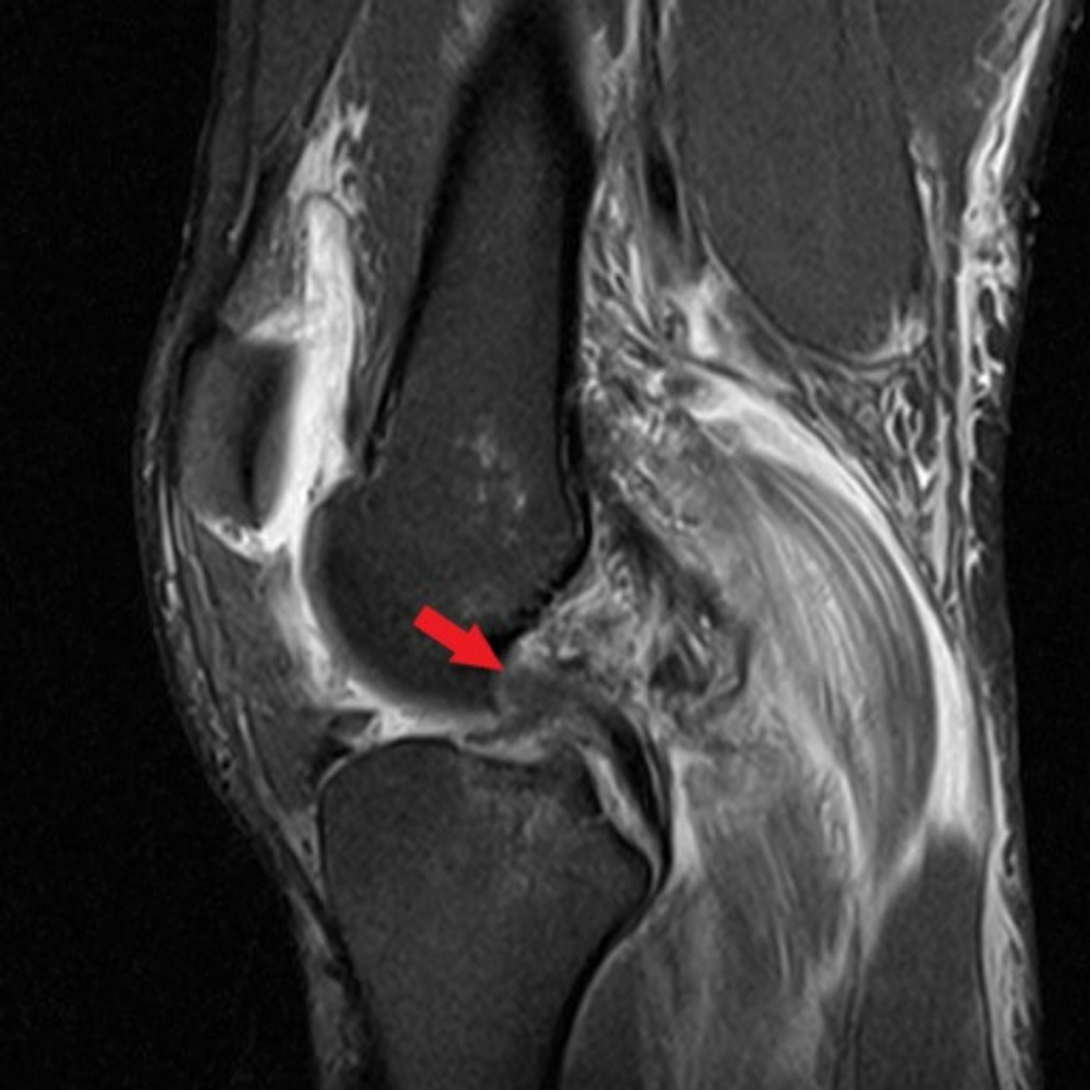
Sagittal T2-weighted MRI of the left knee in patient 2 Sagittal T2-weighted MRI of the left knee demonstrating an increased signal in the proximal aspect of the PCL (red arrow), suggesting possible PCL avulsion injury. This was supported by a physical examination and further confirmed intraoperatively.
